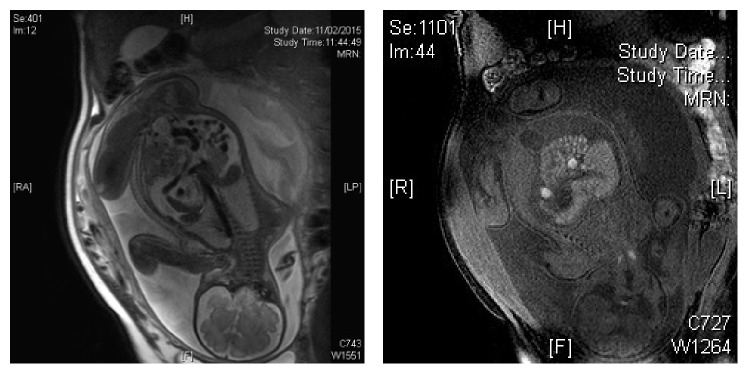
Fetal pelvic MRI showed T1 and T2 isointense soft tissue mass.
